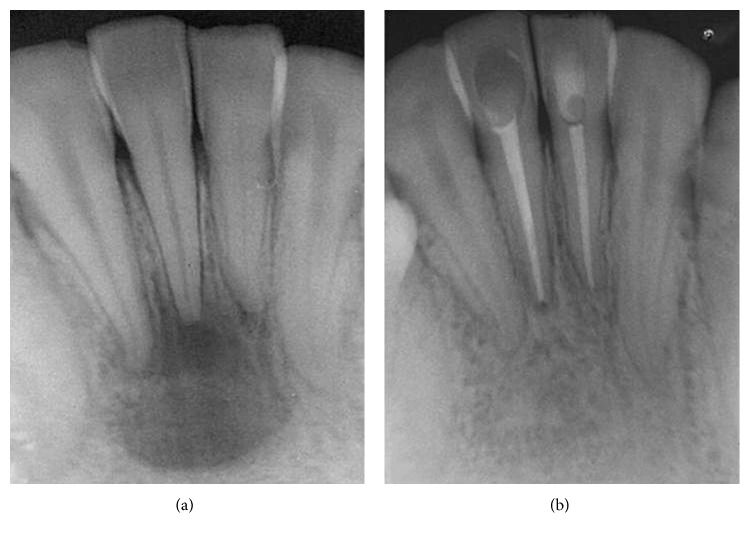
Healing AP on teeth 31, 41, and 42 with a large periapical lesion (PAI = 5). (a) Preoperative view. (b) The lesion is considered healed after 2 years (PAI = 1).
